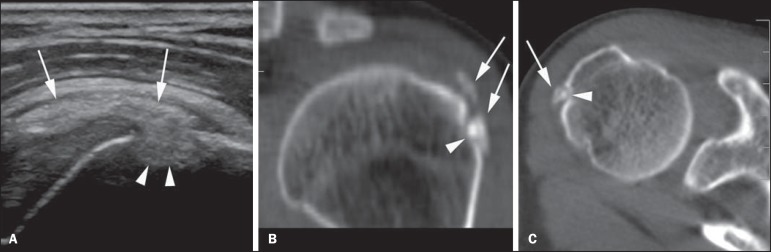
Male, 56-year-old patient. A: Ultrasonography in the long
